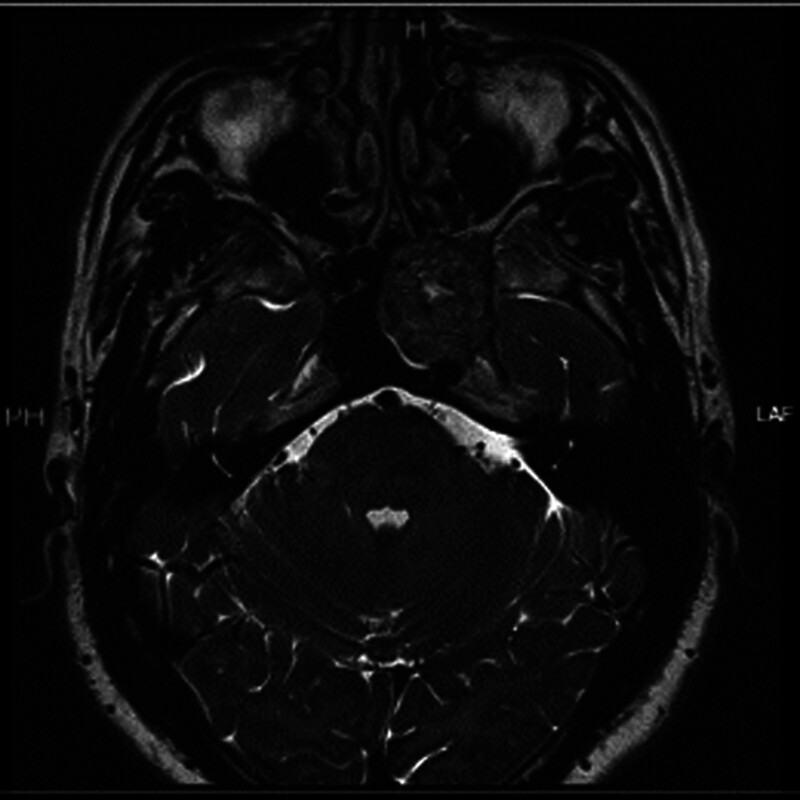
A 54-year old male patient with skull base schwannoma. Sinus MRI horizontal view. MRI = magnetic resonance imaging.
